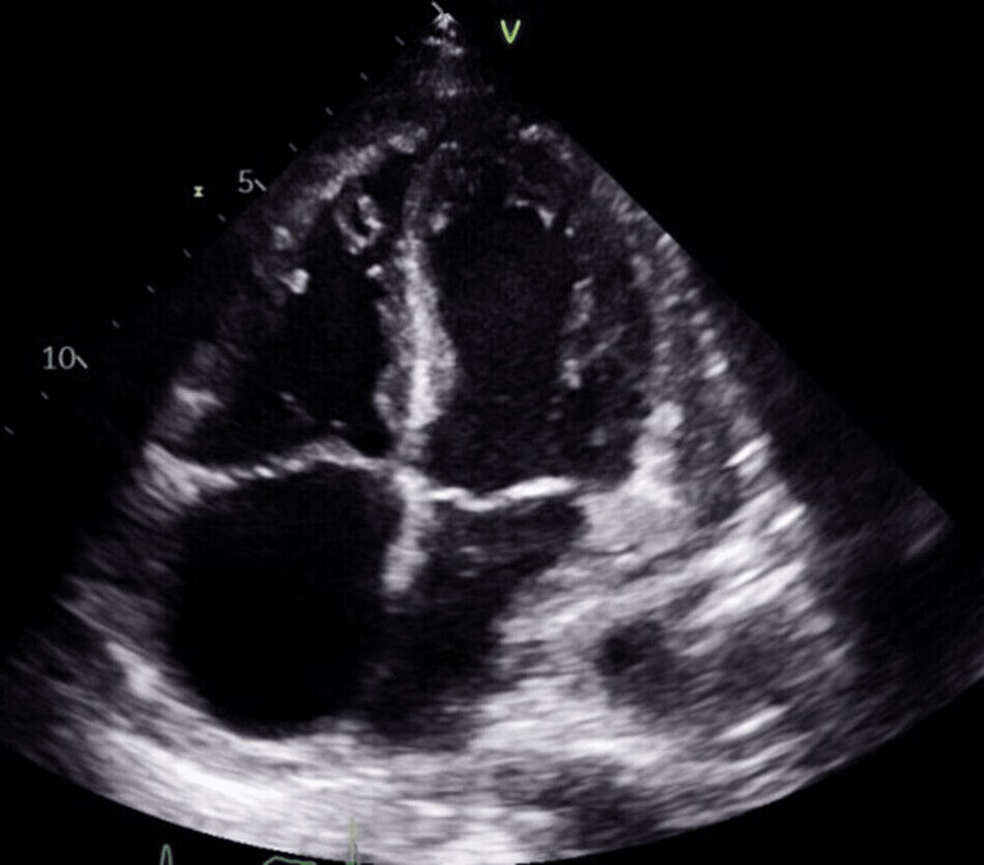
Postoperative TTE showing resolution of the prior pericardial effusion TTE - transthoracic echocardiogram
